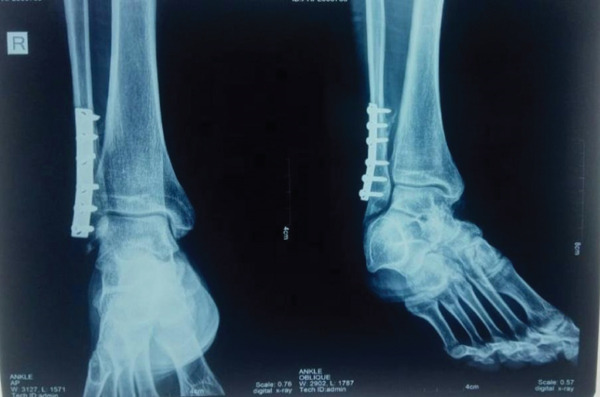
Anteroposterior and mortise plain radiographs of right ankle showing fracture alignment with a semitubular plate in situ post‐4 months of surgery.
